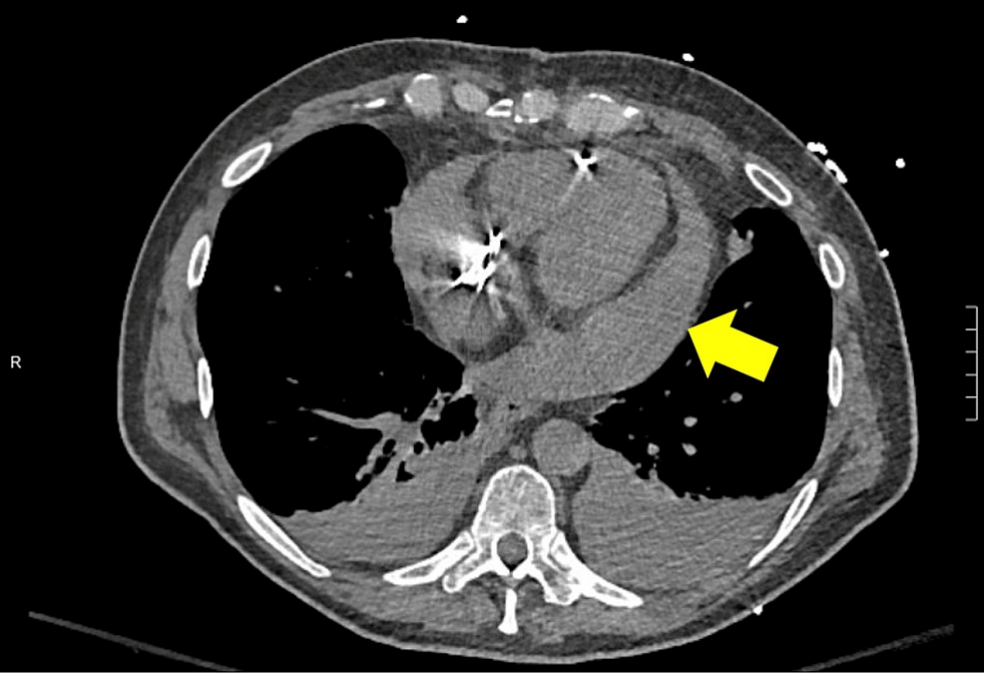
CT scan of the chest without contrast demonstrates dense moderate pericardial effusion (yellow arrow) with Hounsfield unit value consistent with acute hemopericardium.
